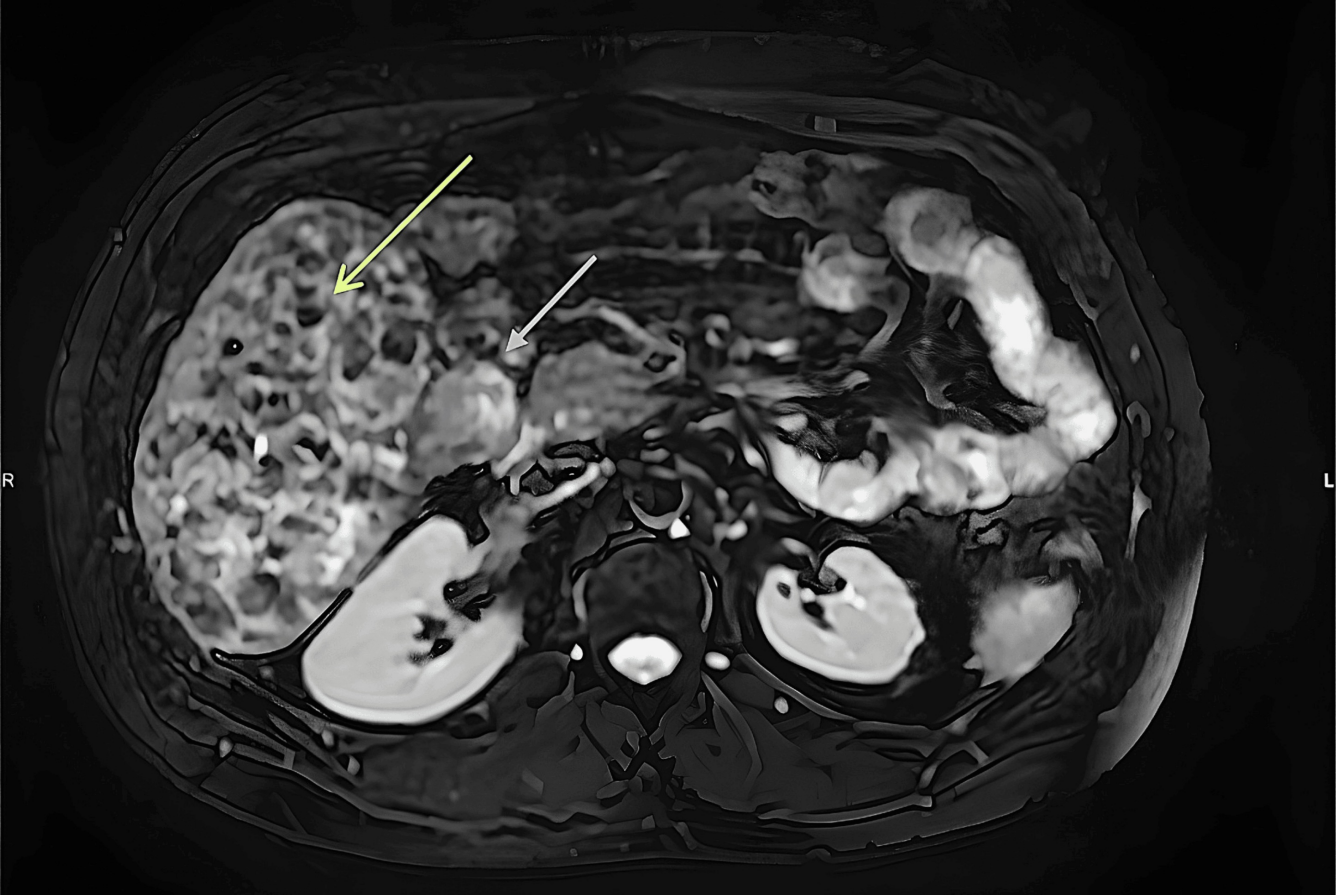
Non-contrast whole-body MRI (axial T2-weighted through the upper abdomen) Showing a 15 cm heterogeneous exophytic liver lesion (shown in yellow arrow) with peripheral nodules, the largest being 4 cm, arising from the liver, in contact with the duodenum and right kidney and close to the inferior vena cava (shown in white arrow) MRI: magnetic resonance imaging
